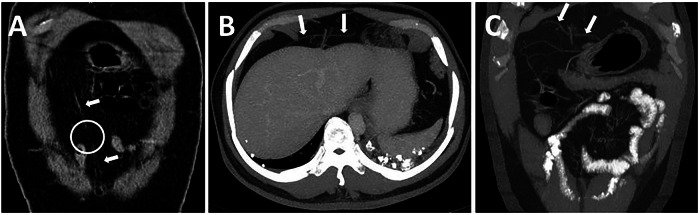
A 75-year-old male patient without a known surgical history underwent a CT scan to investigate epigastric pain. The coronal plane CT image shows a defect (circle) in the middle part of the FL (arrows). B, C FL defect in a 34-year-old male patient without a known surgical history. Axial (B) and coronal (C) plane contrast-enhanced CT images show an omental vein penetrating the FL, indicating the FL defect at this site (asymptomatic omental herniation through the FL)
